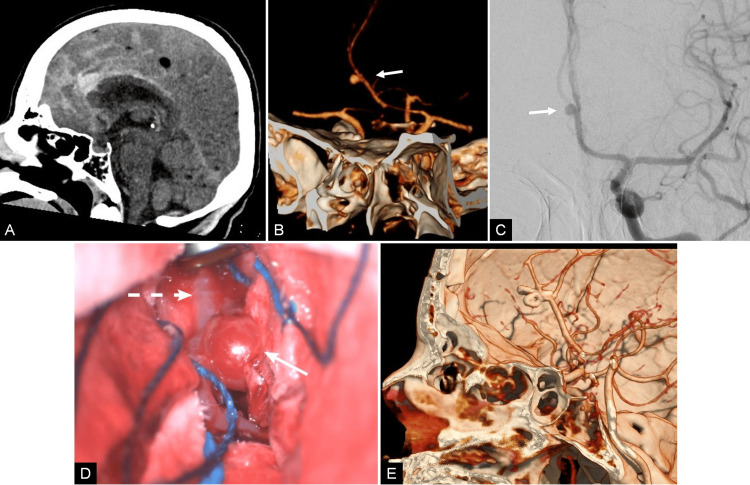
Pre- and postoperative radiological studies of case 4. (A) CT sagittal section showing diffuse hyperdense images in the pericallosal cistern and interhemispheric fissure. (B) Preoperative CTA with 3D reconstruction and (C) left carotid angiography in anteroposterior projection showing a saccular distal azygos aneurysm (arrow). (D) Surgical view depicting temporary clipping of the azygos ACA (dashed arrow) and dissection of the AIHF depicting a distal saccular aneurysm (arrow). (E) Postoperative CTA with 3D reconstruction demonstrates the exclusion of the aneurysm.
